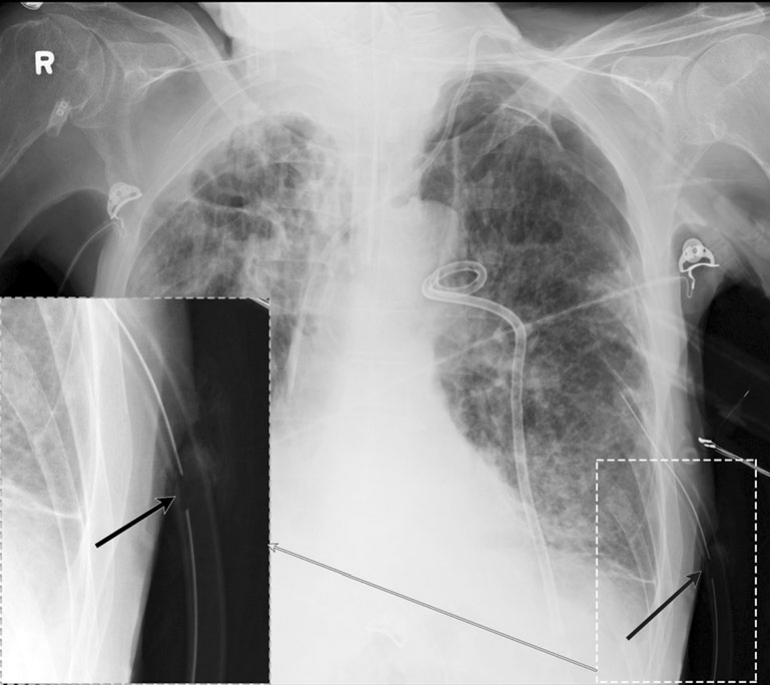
An 88-year-old male with underlying COPD and bullous emphysema presented with a spontaneous left pneumothorax. Despite subsequent chest tube and anterior pleural catheter placement, left pneumothorax persisted. Upon careful review of the radiograph, side hole of the left chest tube was outside the pleural cavity (*black arrow*) and communicated with the atmospheric air
